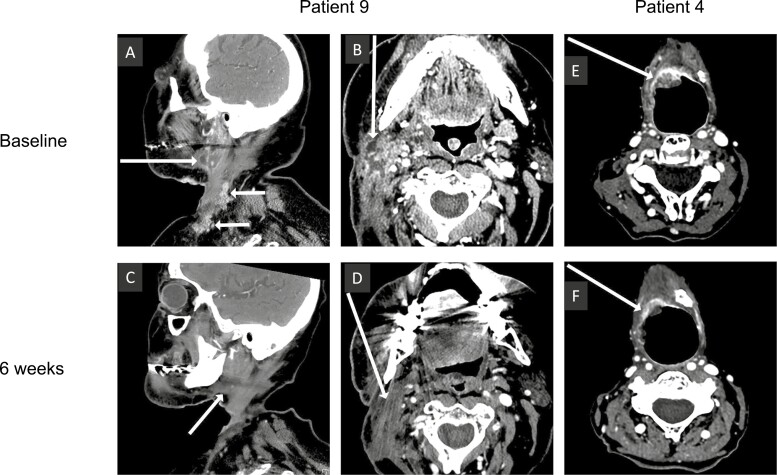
Responses to bimiralisib. Computed tomography images from patient 9 (A-D) and patient 4 (E, F) at baseline (A, B, E) and after 6 weeks of therapy (C, D, F). White arrows indicate head and neck squamous cell carcinoma tumor location.
